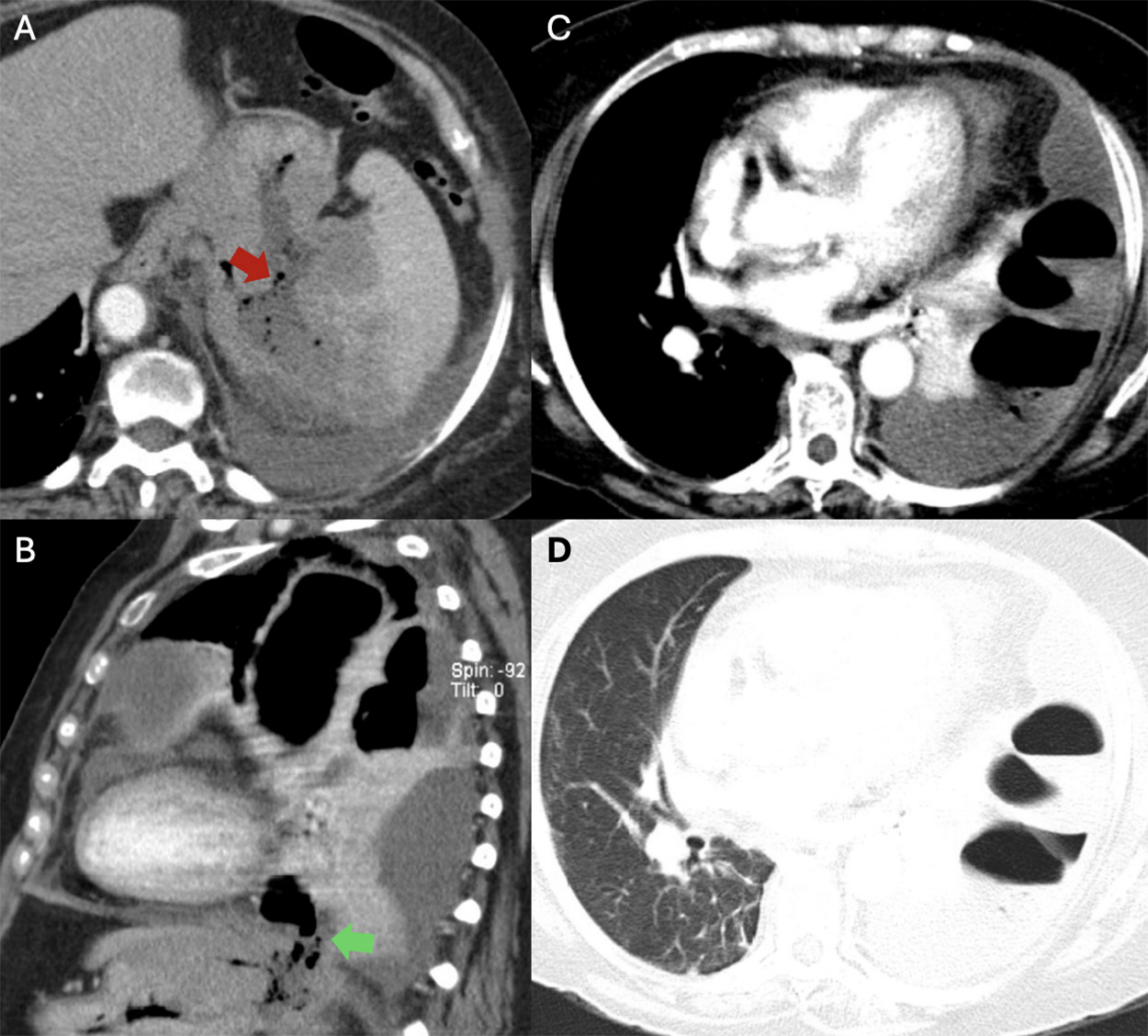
Contrast‑enhanced CT images demonstrate a gastrosplenic fistula (red arrow in A) and a diaphragmatic defect (green arrow in B), resulting in leakage of gastric contents into the pleural cavity and the development of a multiloculated hydropneumothorax (C and D).
